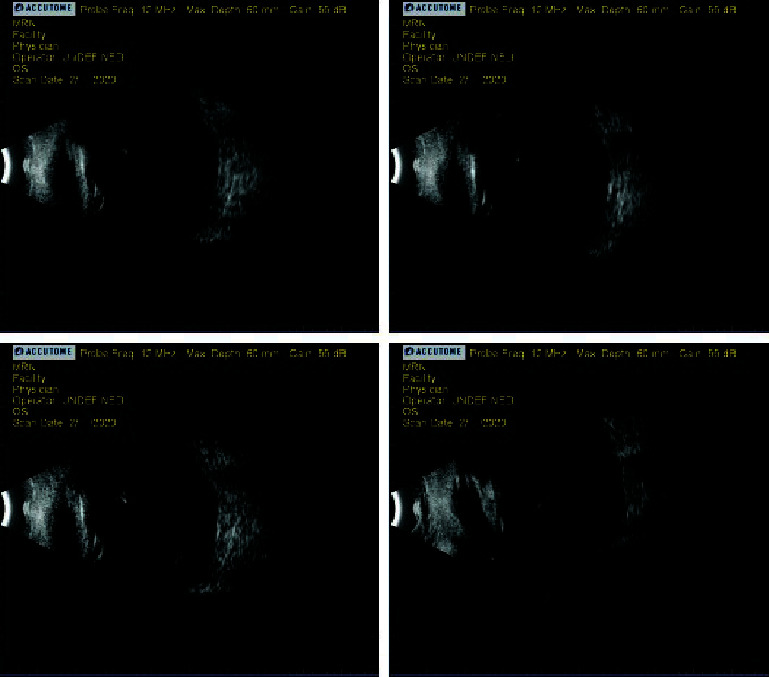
Ultrasound B-scan from postop day 1 (following vitrectomy). Mild vitreous haziness but no choroidals present.
